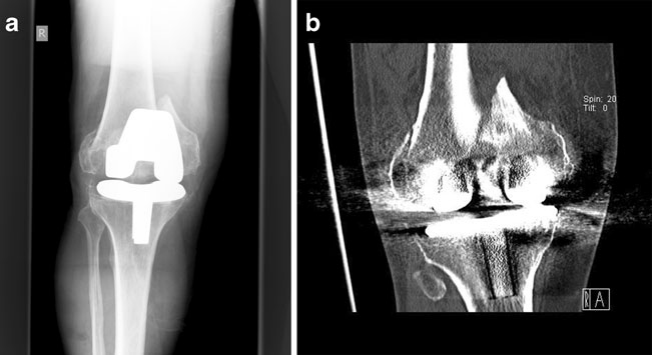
Preoperative X-ray (a) and CT (b) view of a partially loose PPF type Rorabeck III, OTA 33 C1; by Steffen Ruchholtz
